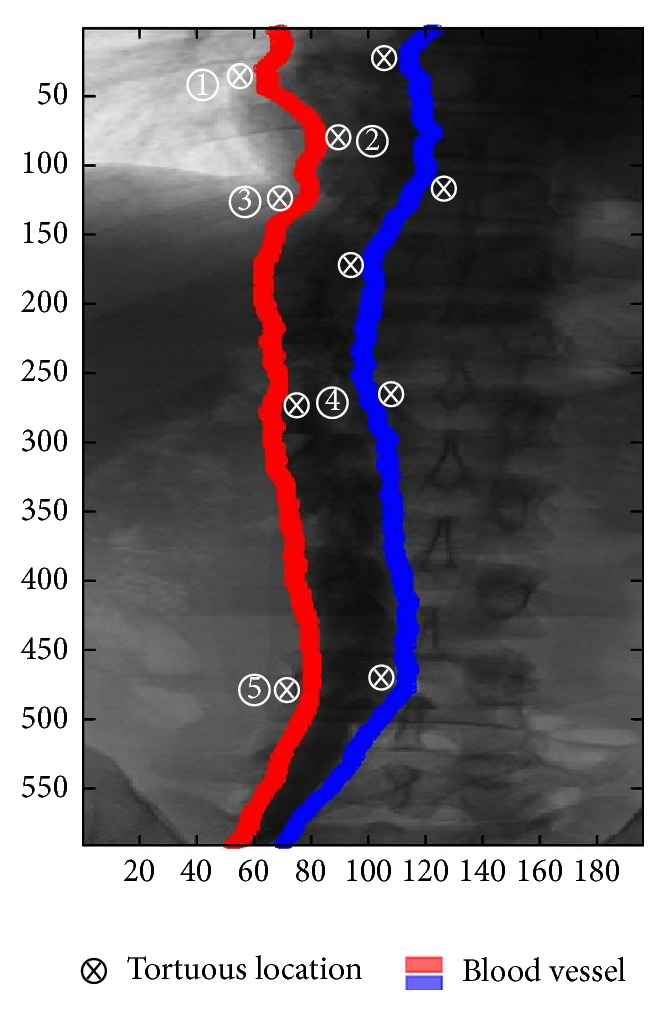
X-ray image, the frontal view of human body in *x*-*y* plane, vessel trajectories as red color and blue color.
